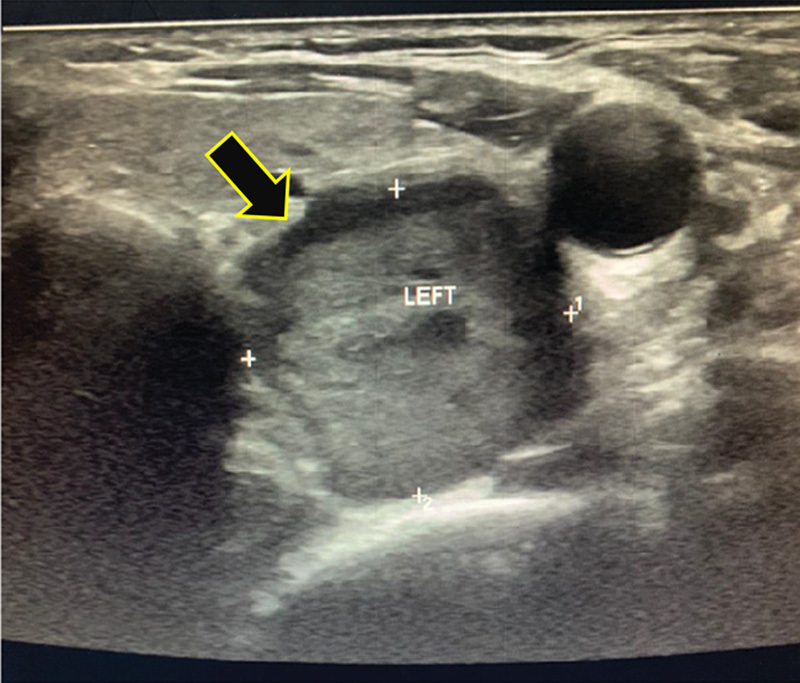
Neck ultrasound showing a hypoechoic, well-defined, heterogeneous, and vascularized lesion measuring 1.9×1.7 cm, seen posterior to the left thyroid lobe (arrow).
